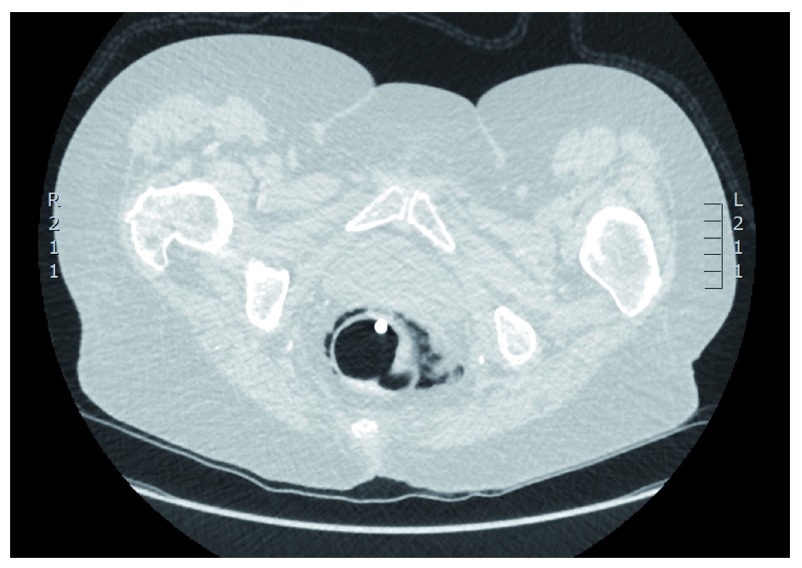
CT colonography depicting contained rectal perforation. The Foley catheter tip is seen in rectum as well as localized peri-rectal air.
